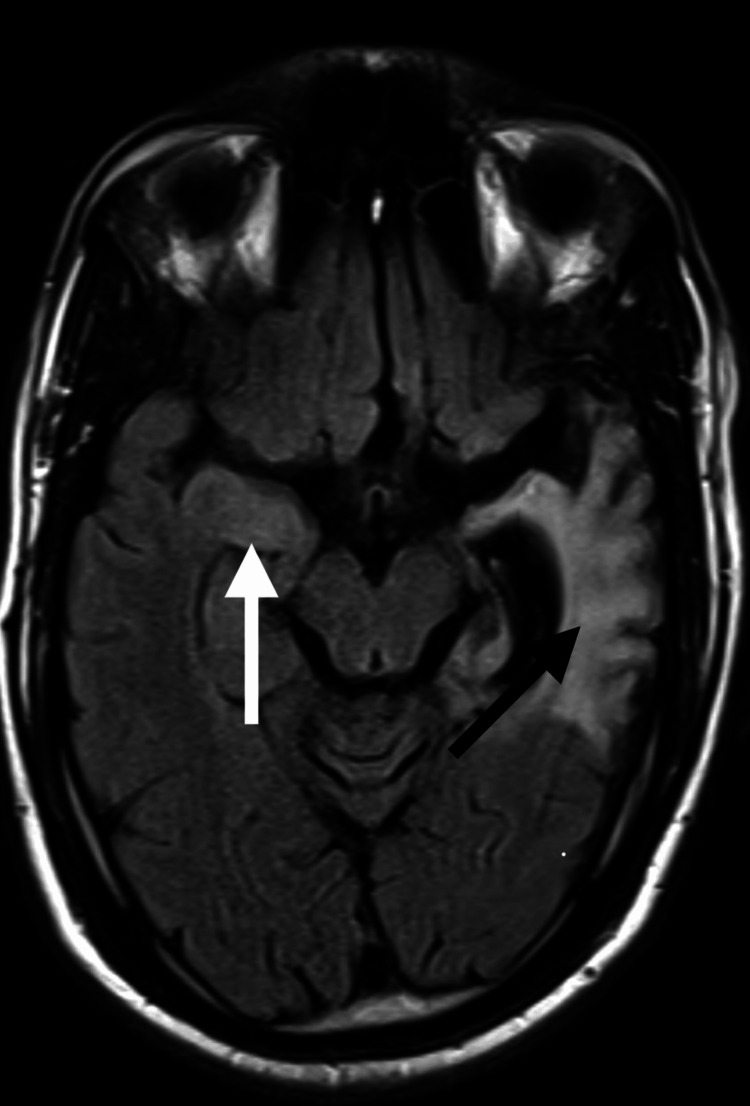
MRI brain axial FLAIR image taken on January 9, 2025, showing left mesial temporal atrophy with ex vacuo hydrocephalus (black arrow) and right mesial temporal hyperintensity (white arrow). FLAIR: Fluid-attenuated inversion recovery.
